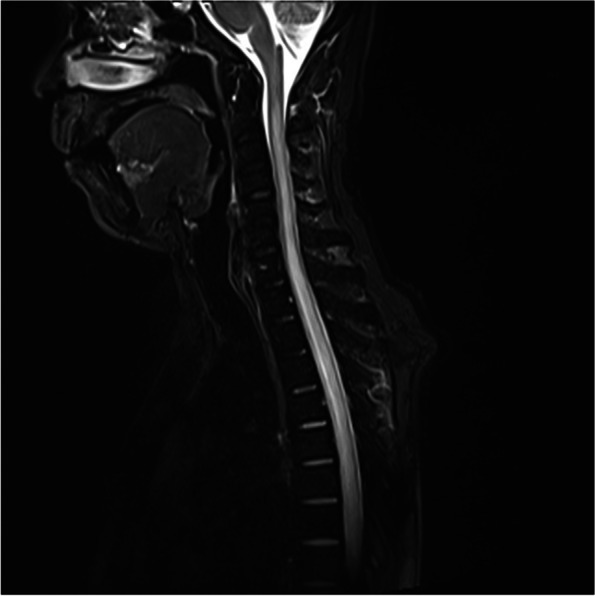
T2W fat supressed MRI spine shows T2 high signal extending from cervico medullary junction to the thoracic spinal cord
